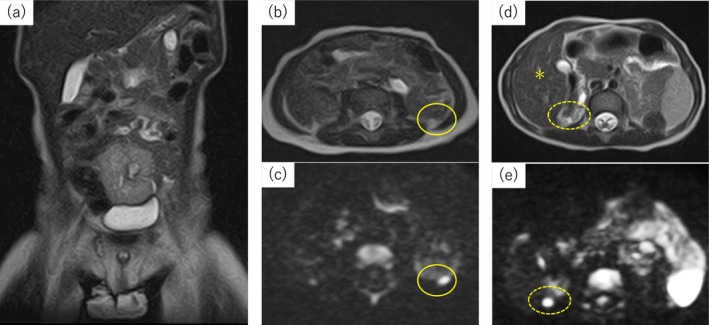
Magnetic resonance imaging (MRI) at 1 year and 2 months (a–c) and 4 years and 3 months of age (d, e). (a) T2‐weighted imaging, coronal section. There are no testes in the inguinal rings. (b) T2‐weighted imaging, axial section and (c) diffusion‐weighted imaging, axial section. The left testis is seen immediately caudal to the spleen (circle), but no right testis is seen. (d) T2‐weighted imaging and (e) diffusion‐weighted imaging show the structure (dotted circle) immediately caudal to the liver (asterisk), thought to be the right testis.
